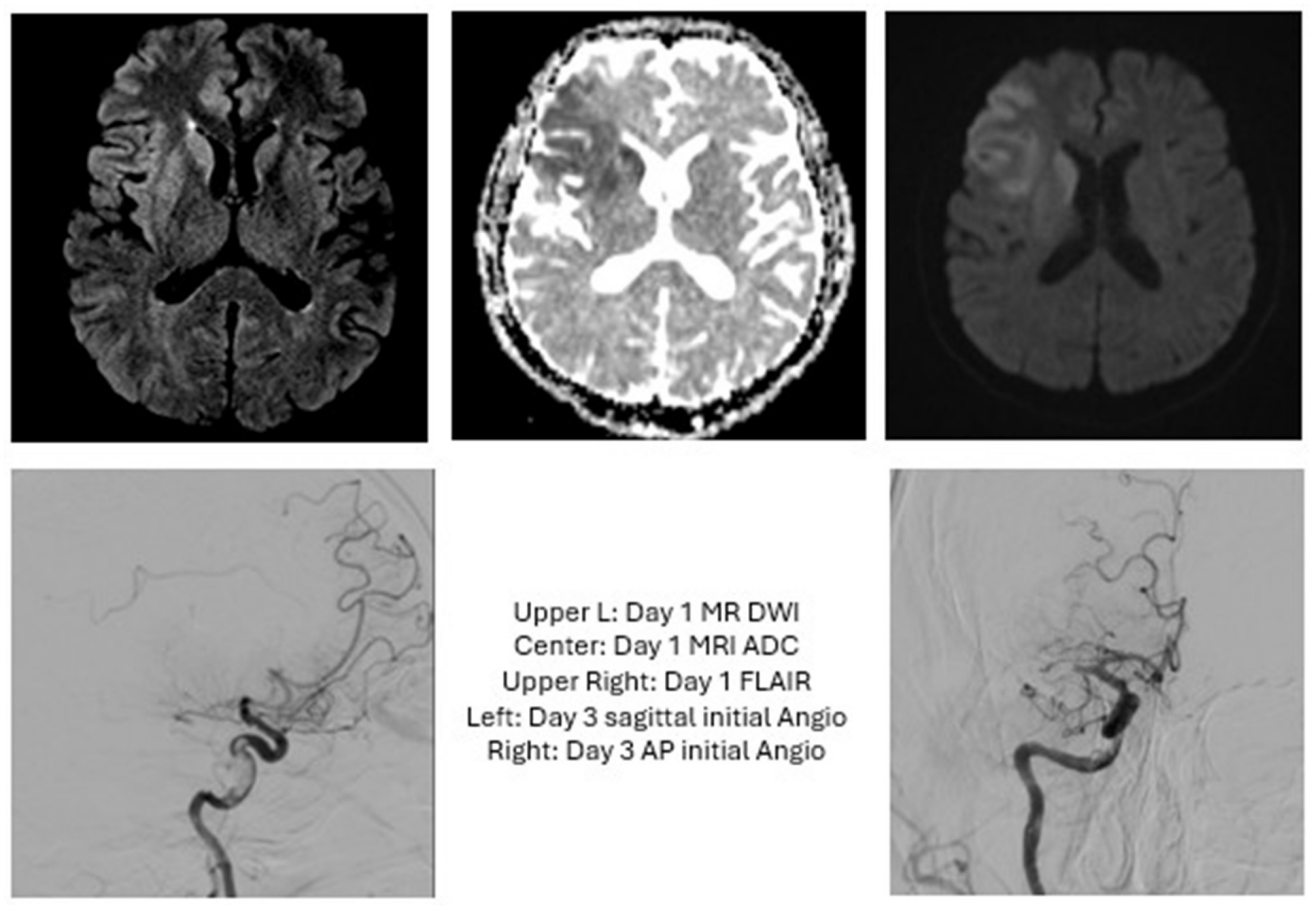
Case 1: The patient presented with an initial NIHSS of 7 and a R ICA occlusion but improved following administration of tPA. Heads Up testing in the IR suite revealed no further worsening, so MT was deferred. Three days later, the patient acutely worsened with NIHSS of 10 and an MRI showing R MCA occlusion. MT was performed with TICI 2C. Post-proceduraly, this patient required decompressive hemicraniectomy and was discharged with a tracheostomy and G-tube.
